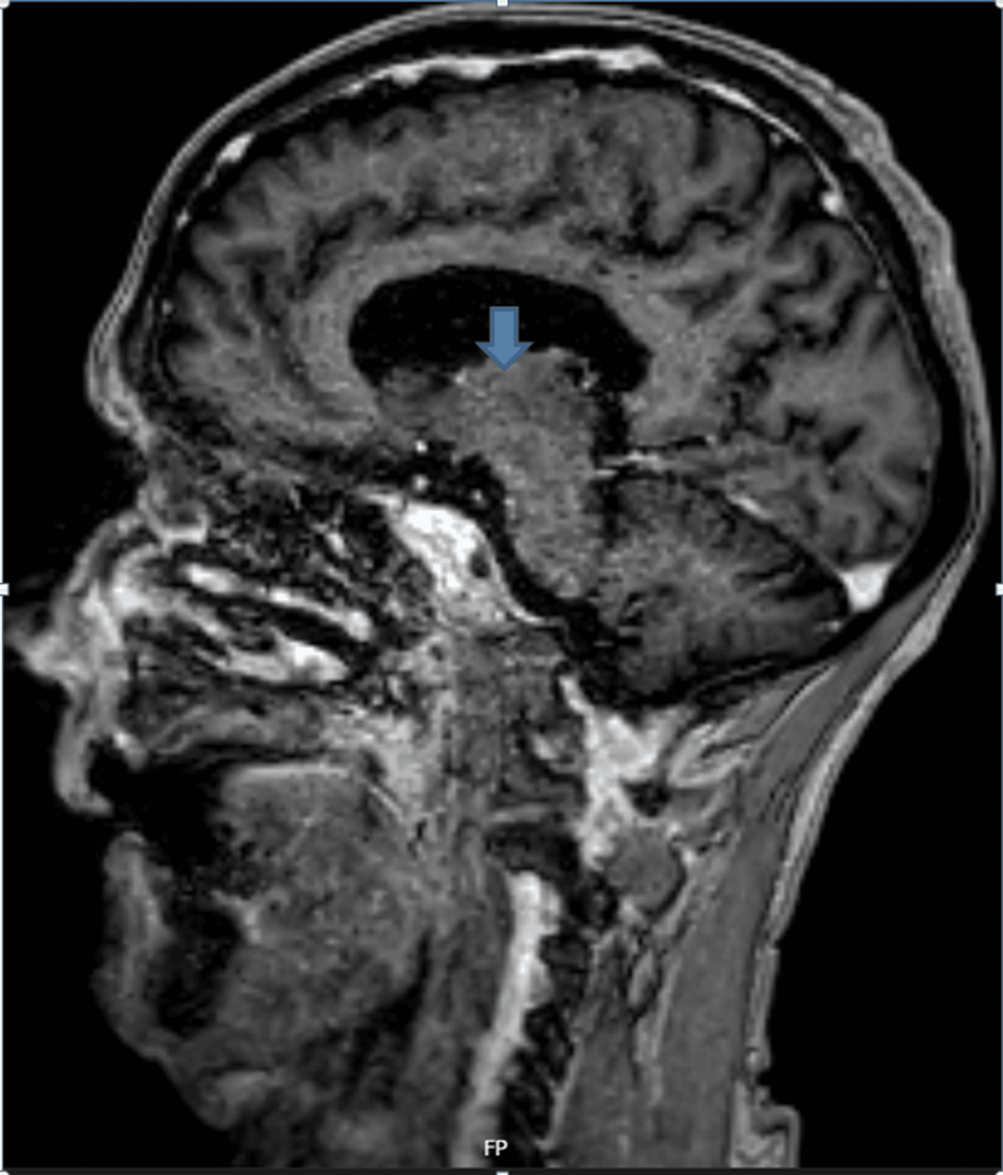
MRI sagittal section showing septocallosal white matter lesion (arrow).
